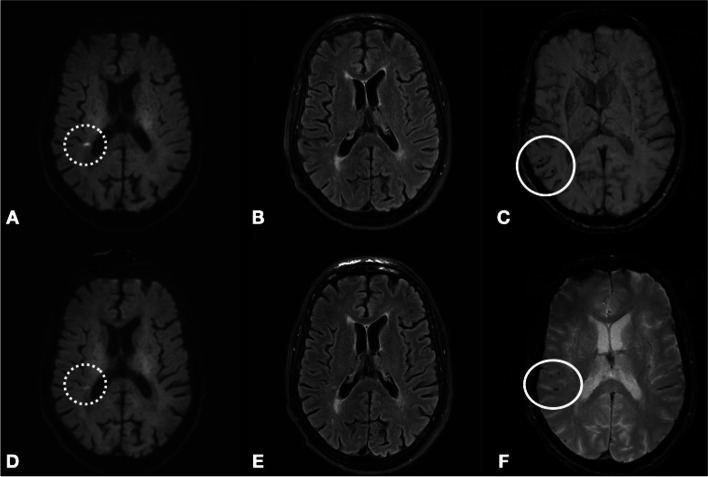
Diffusion-weighted imaging (DWI). Fluid attenuated inversion recorvery (FLAIR). Susceptibility weighted imaging (SWI)/T2* on day 0 (A-C) and 14 (D-F). DWI sequence (A and D) shows a hyperintense lesion in relation to the right lateral ventricle (dotted circle). SWI reveal multiple cortical microbleeds in the same area (solid circle)
